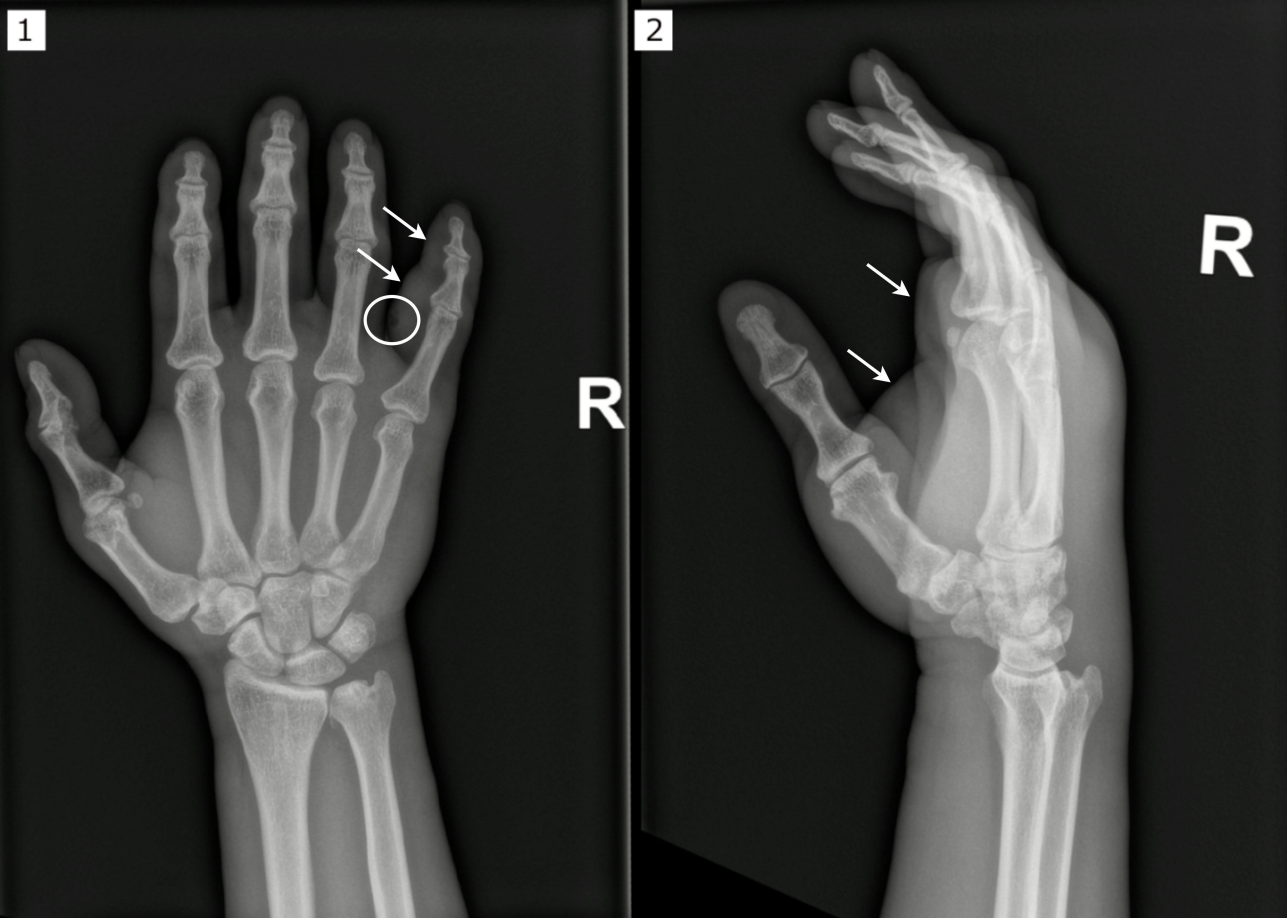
X-ray of the patient’s right hand in (1) posteroanterior and (2) lateral projections with soft tissue swelling and subcutaneous emphysema on the palmar aspect of the fifth proximal phalanx. The circle demonstrates where the subcutaneous emphysema is located, while the arrows indicate soft tissue swelling.
